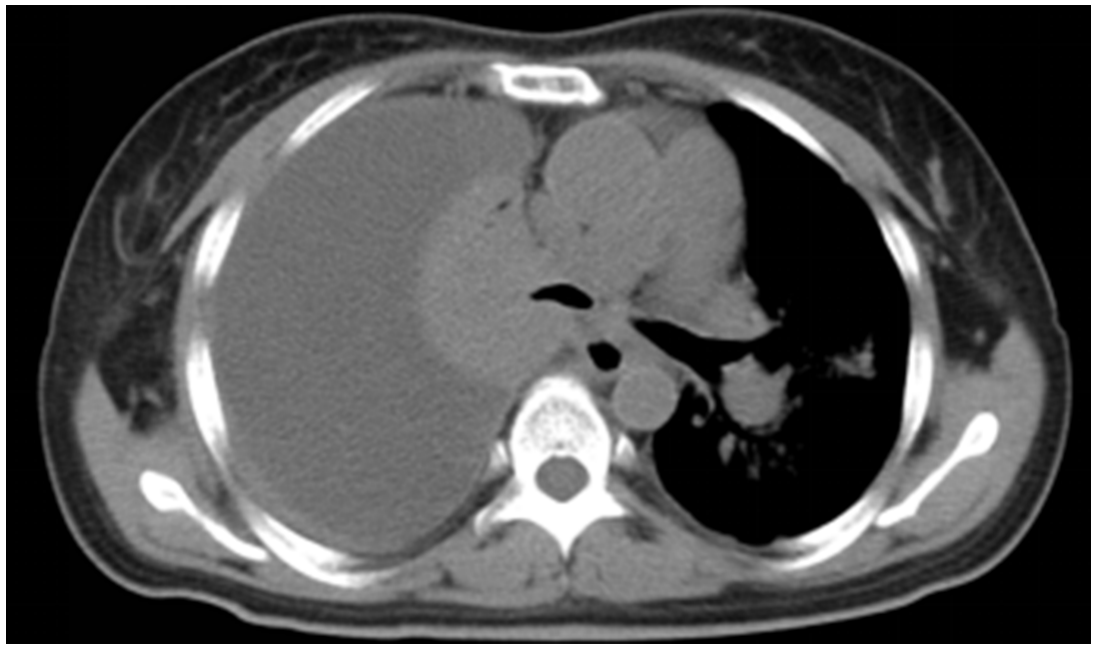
Six months after treated with Gefitinib, a repeat CT scan showed the disease progressed
